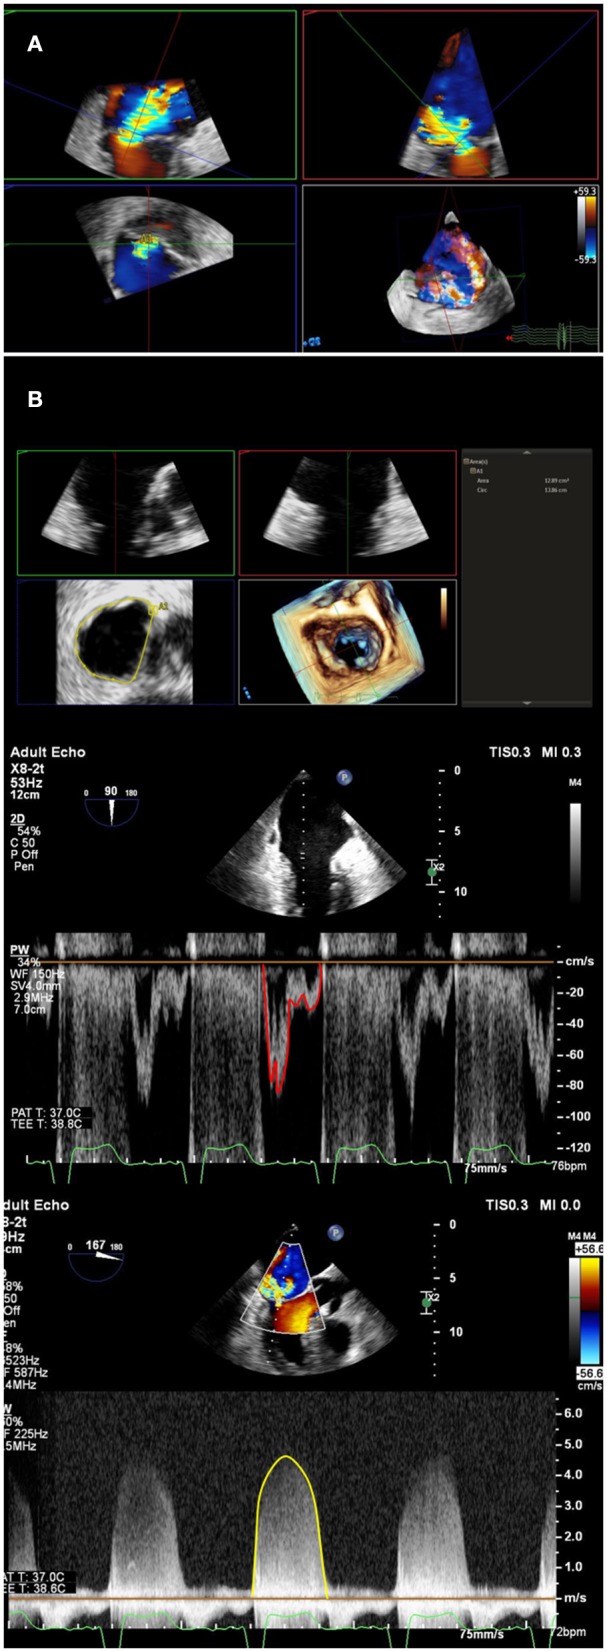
Multi-parametric assessment of mitral regurgitation. In addition to qualitative color Doppler grading, multiple methods of mitral regurgitant quantification should be used. (A) A multiplanar reconstruction of 3-Dimensional echocardiographic color Doppler can be used to directly planimeter vena contracta area. A double-oblique method is used to align long-axis images (upper left and right), and directly planimeter vena contracta area (lower left). (B) Mitral valve inflow stroke volume, which includes mitral regurgitant volume, can be calculated by multiplying mitral annulus area (top panel) by pulsed-wave velocity time integral at the annulus level (middle panel). Left ventricular or right ventricular outflow tract calculated stroke volume, in the absence of aortic or pulmonic insufficiency, can be subtracted from mitral stroke volume to obtain regurgitant volume. Regurgitant volume can be divided by mitral regurgitation continuous-wave Doppler velocity time integral (bottom panel) to calculate quantitative Doppler derived regurgitant orifice area.
